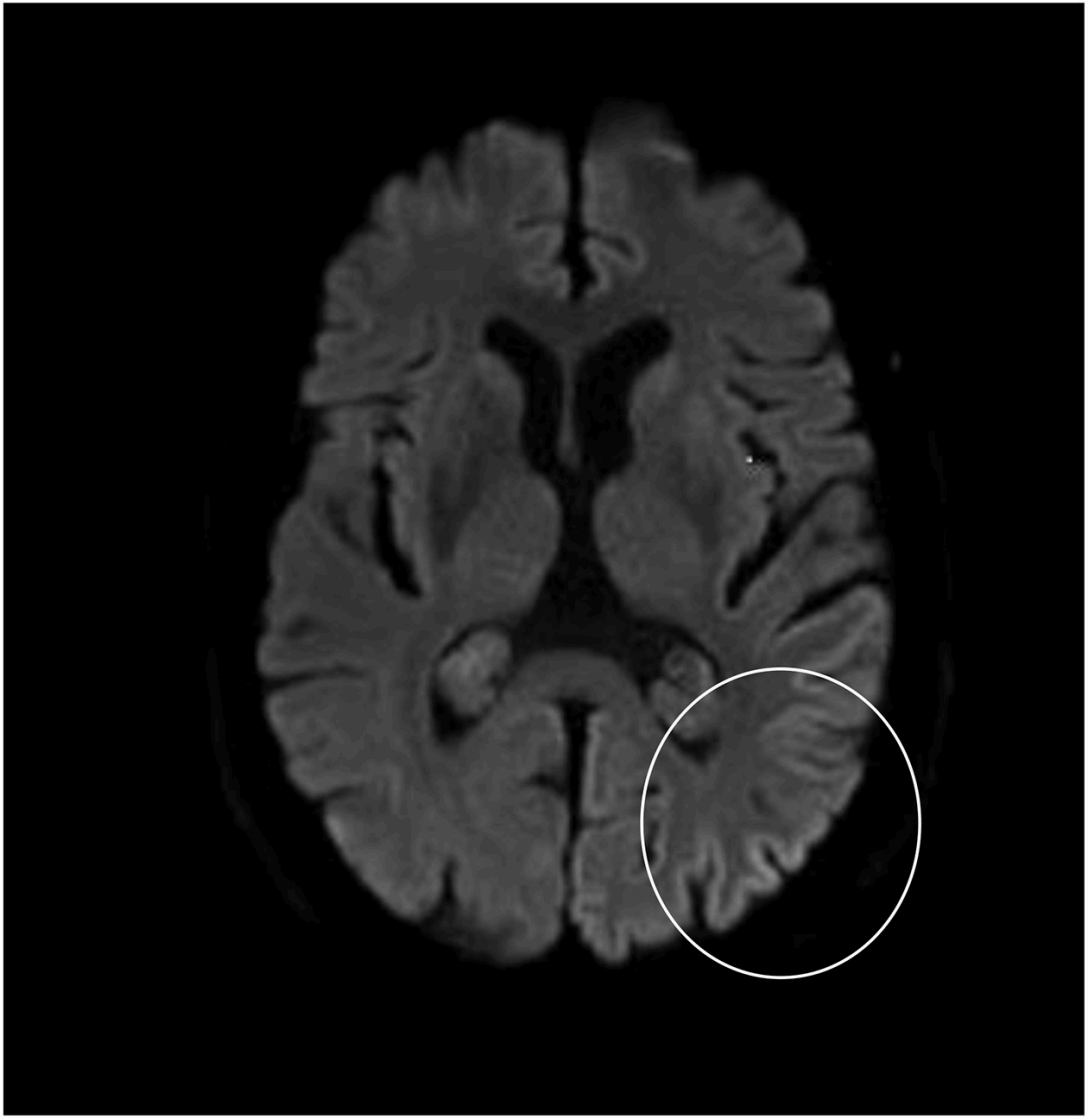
High signal intensity of the left parieto-occipital cortex on diffusion-weighted MRI (DWI; b = 1,000).
